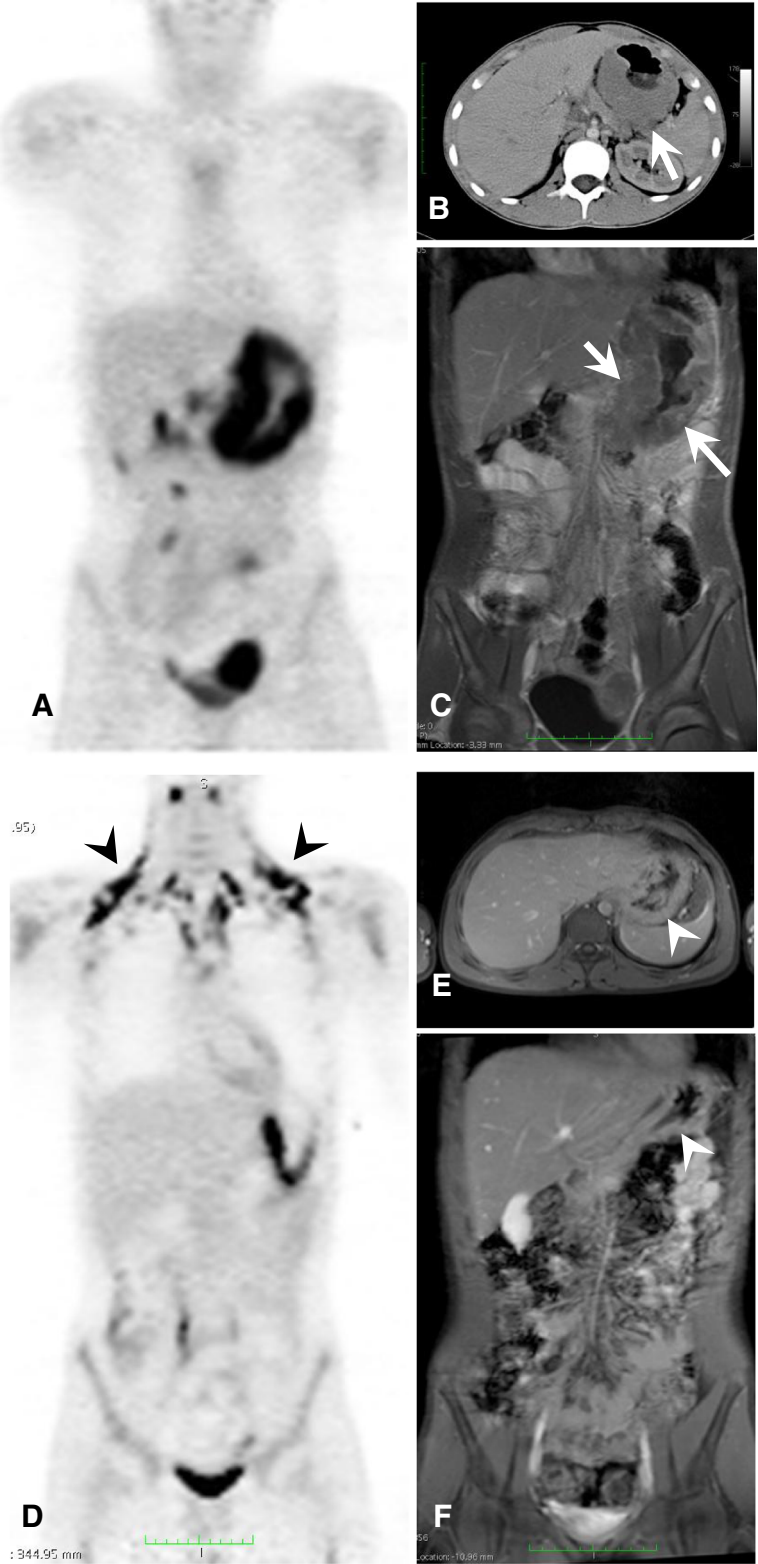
Fourteen-year-old boy suffering from Burkitt-lymphoma (Table2, patient 15). The lymphoma was primarily localized in the stomach (A, B, C). Initial SUVmax was 21.5 and decreased to 10.6 at interim time point (A and D; *Δ*SUVmax, 50.7%). Also, in the visual iPET analysis (D), the patient was judged to be positive (please note activation of brown adipose tissue at interim marked with black arrow heads (D). Using CIM, a decrease of 100% between baseline (arrows in B and C) and interim time points was shown (arrow heads in E and F). No further measurable disease was detected by CIM. Thus, a complete response was indicated by CIM. The patient is in ongoing remission. Therefore, both *Δ*SUVmax and visual iPET analyses were false-positive when compared to true outcome, whereas CIM was true-negative.
